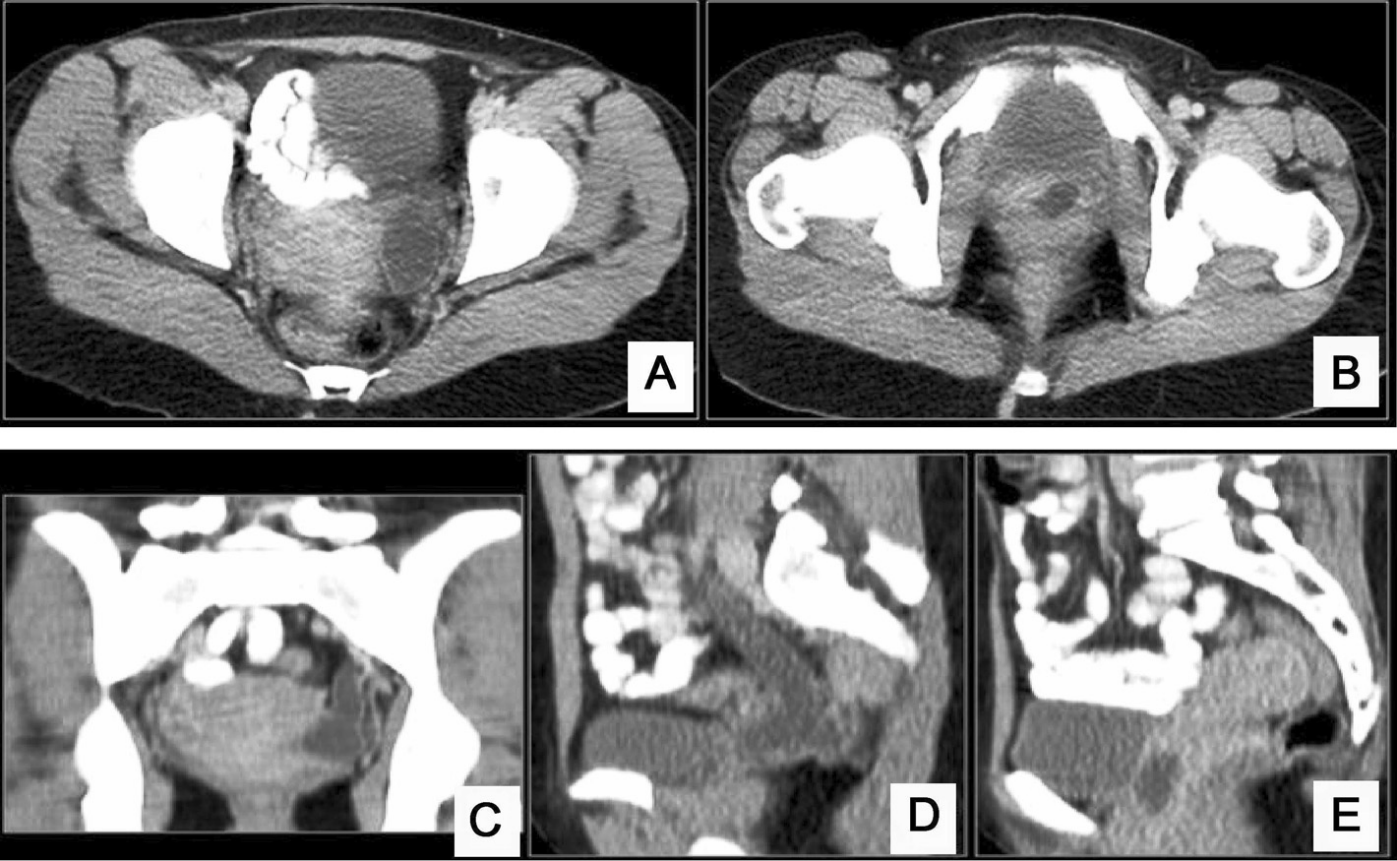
Computed tomography findings. Axial contrast-enhanced computed tomography (CT) images of the pelvis show a well-defined oval-shaped cystic lesion at the left of the uterus body (A) that extends to the anterior wall of the vagina (B). Coronal (C) and sequential sagittal (D, E) contrast-enhanced CT images of the pelvis reveal the tubular morphology of the well-defined cystic lesion at the left of the uterus body (C) that extends caudal to the anterior wall of the vagina (D) and has a configuration that resembles a ureter.
